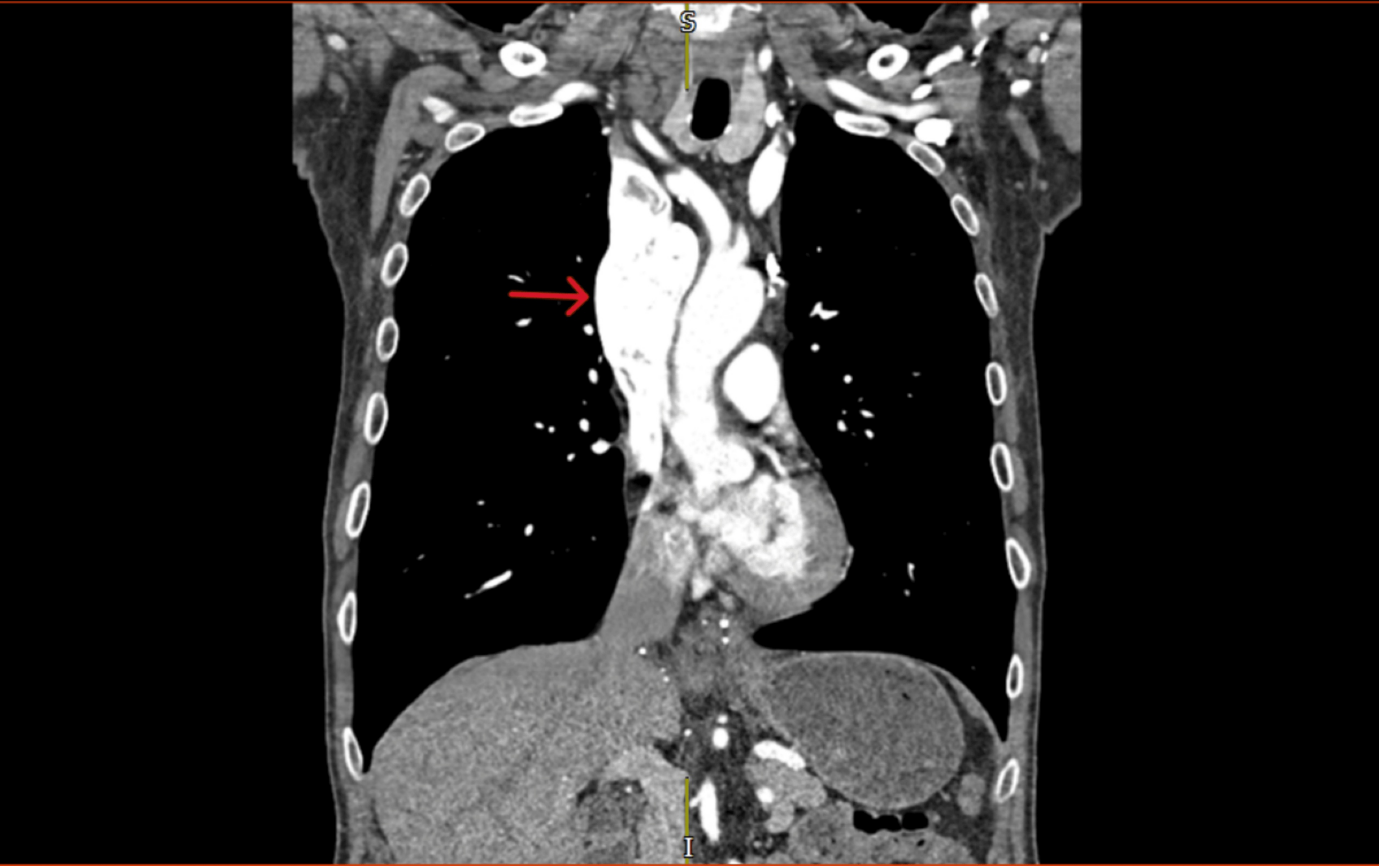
Contrast-enhanced computed tomography image (coronal view) The image is showing the large fusiform superior vena cava aneurysm (red arrow)
